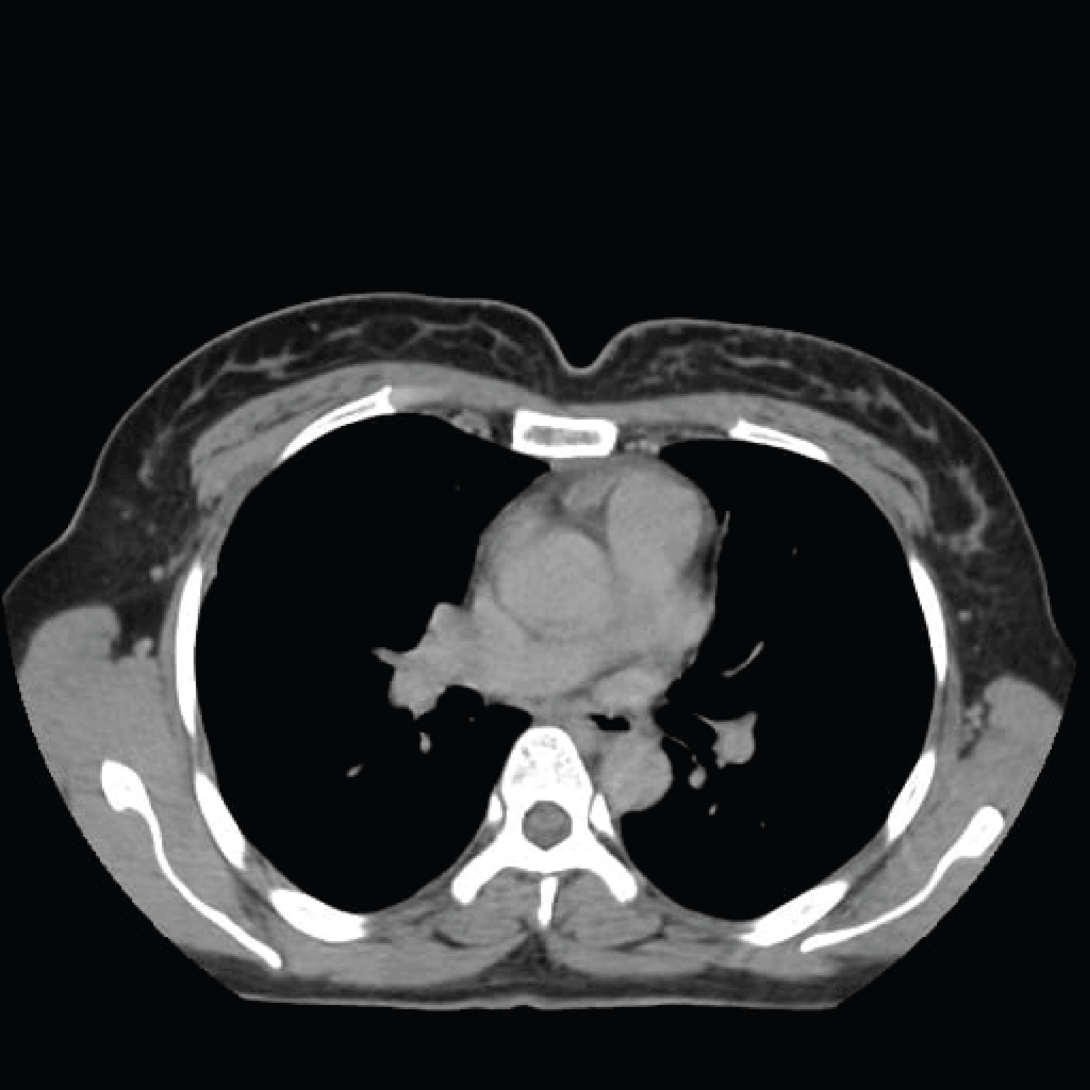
Follow-up axial thoracic CT scan in the mediastinal window demonstrating no evidence of pleural empyema or pericardial effusion
